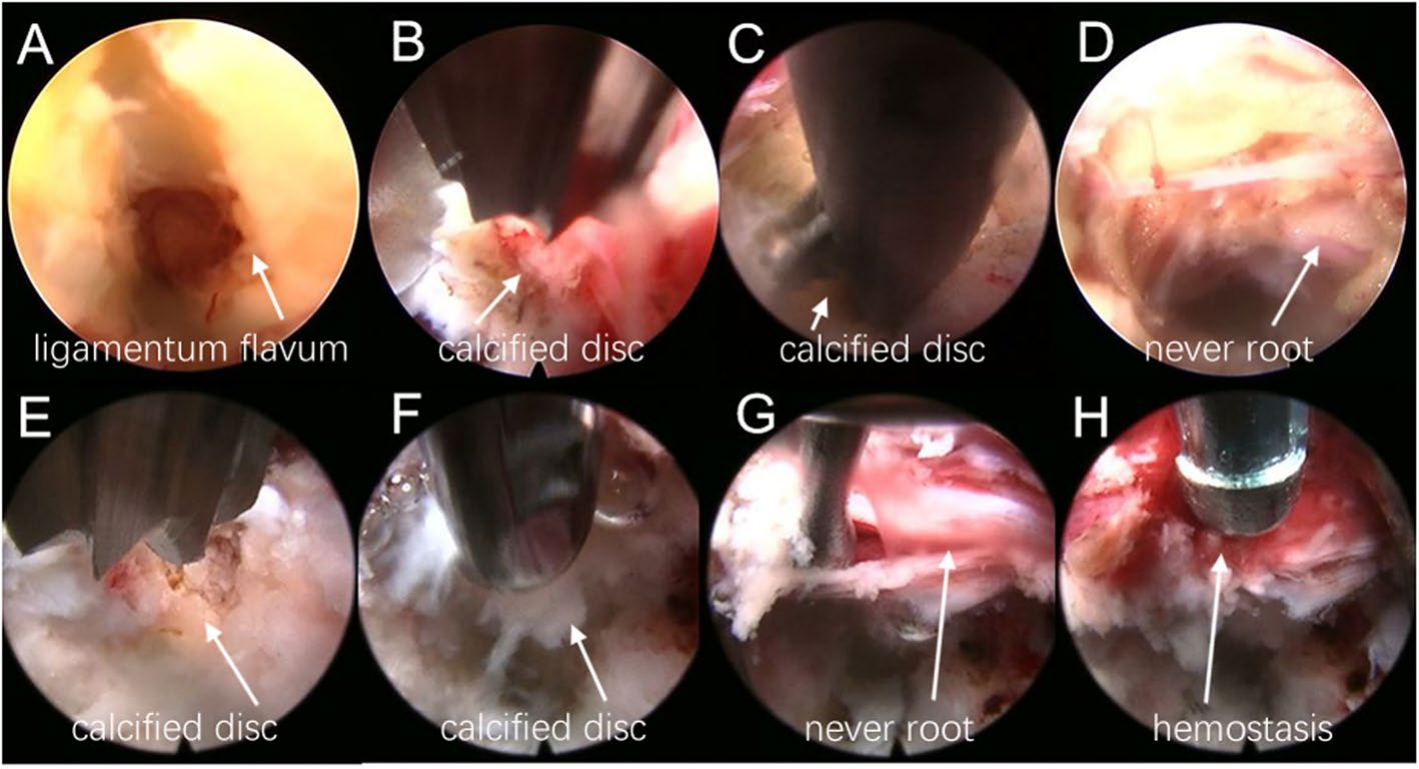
Endoscopic images of PEID (A-D) and PETD (E–H). A The ligamentum flavum was cut by scissors. B The calcified and herniated disc tissue was cut by an ultrasonic osteotome. C The calcified and herniated disc were taken out by a nucleus pulposus forceps. D The nerve root and dural sac were fully decompression. E An ultrasonic osteotome was used to cut the calcified disc. F A nucleus pulposus forceps was used to remove the calcified and herniated disc. G The nerve roots and dural sac were moved. H A bipolar radiofrequency was used to hemostasis
